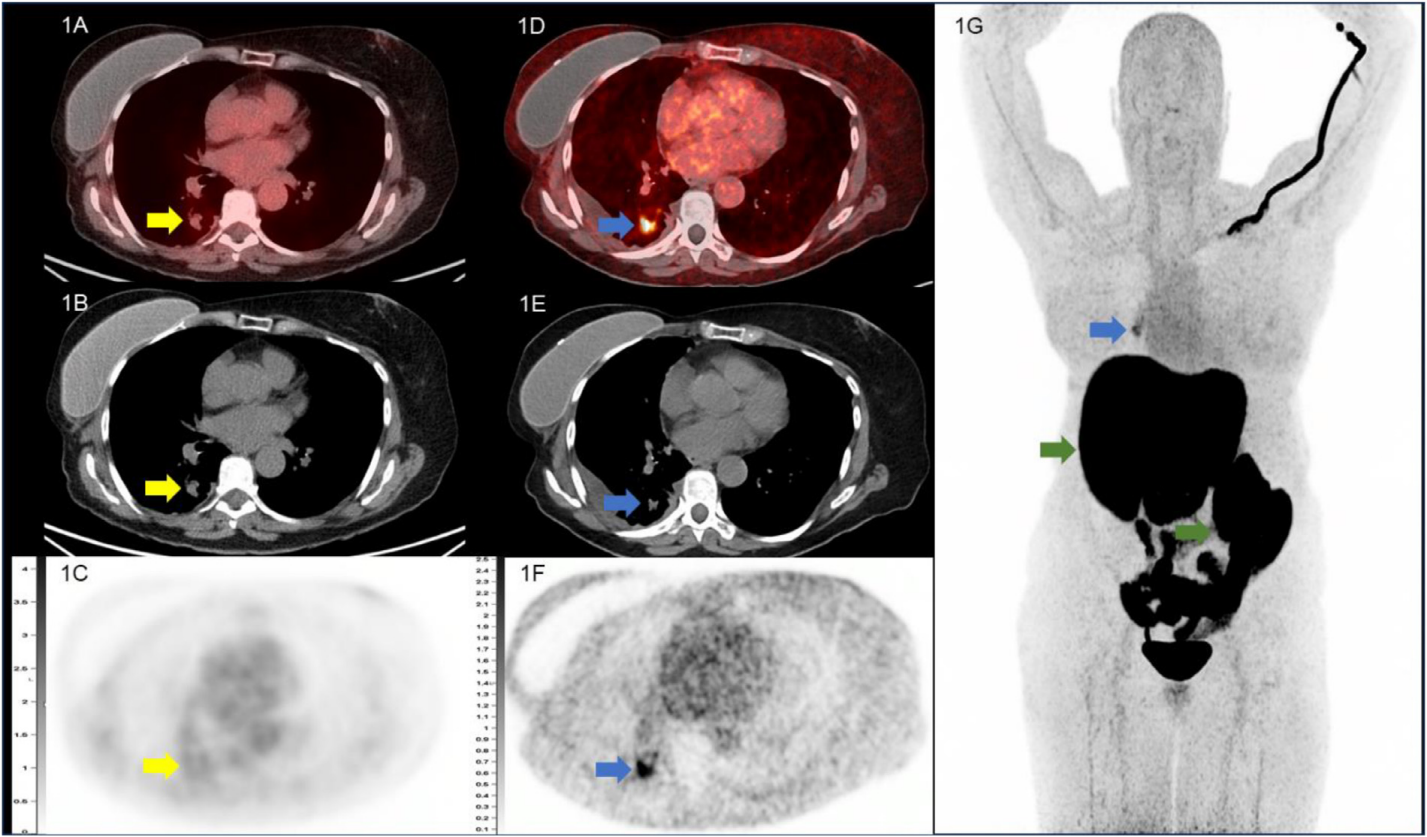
Selected transaxial images from the initial FDG PET/CT and subsequent FES PET/CT in this patient with enlarging pulmonary nodule and prior ER+ breast cancer and pulmonary adenocarcinoma. Transaxial fused FDG PET/CT (1A) CT (1B) and FDG PET (1C) images show a right lower lobe pulmonary nodule with uptake comparable to blood pool with SUVmax 2.5 (yellow arrow). Transaxial fused FES PET/CT (1D) CT (1E) and FES PET (1F) images demonstrate avid uptake in the pulmonary nodule with SUVmax 3.0 (blue arrow). Coronal FES PET maximum intensity projection (MIP; 1G) demonstrates the FES avid pulmonary nodule which was biopsy proved pulmonary adenocarcinoma (blue arrow) and no other abnormal FES avid foci to suggest additional ER+ disease, either from lung or breast primary. Note intense physiological FES uptake in the liver and excretion through the biliary tree into the bowel (green arrow).
